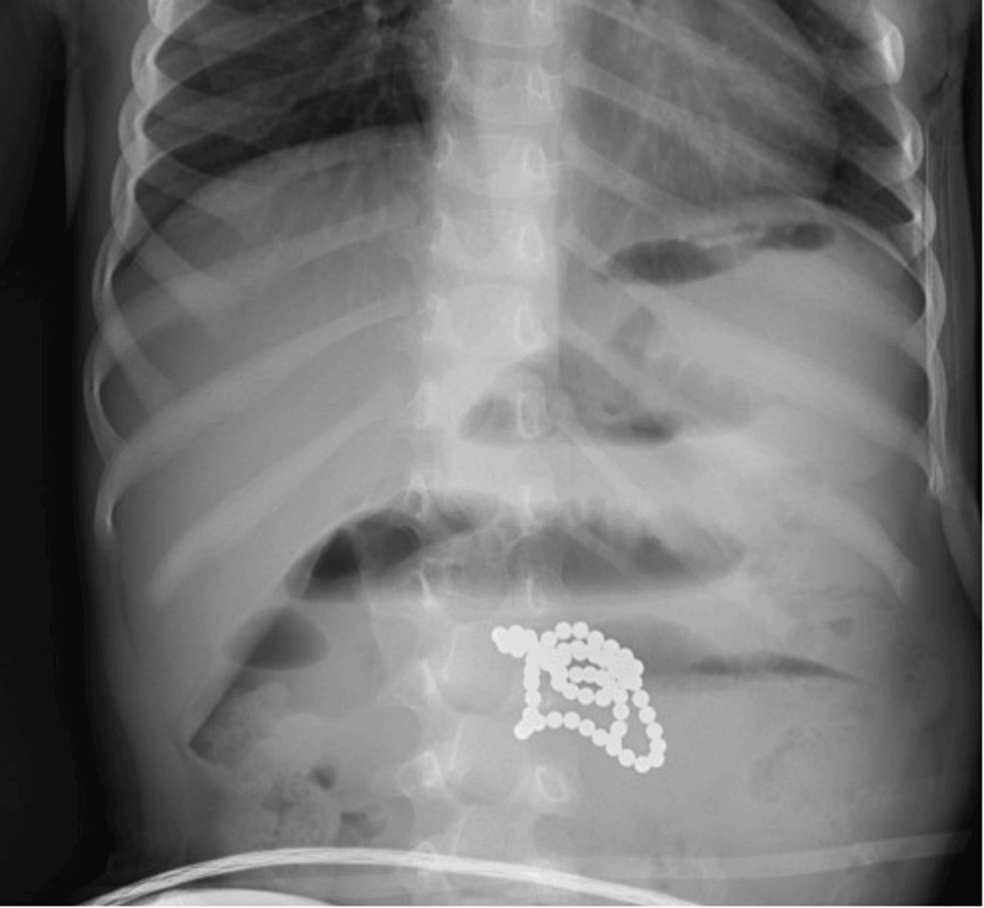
Abdominal X-ray With Multiple Dilated Bowel Loops
